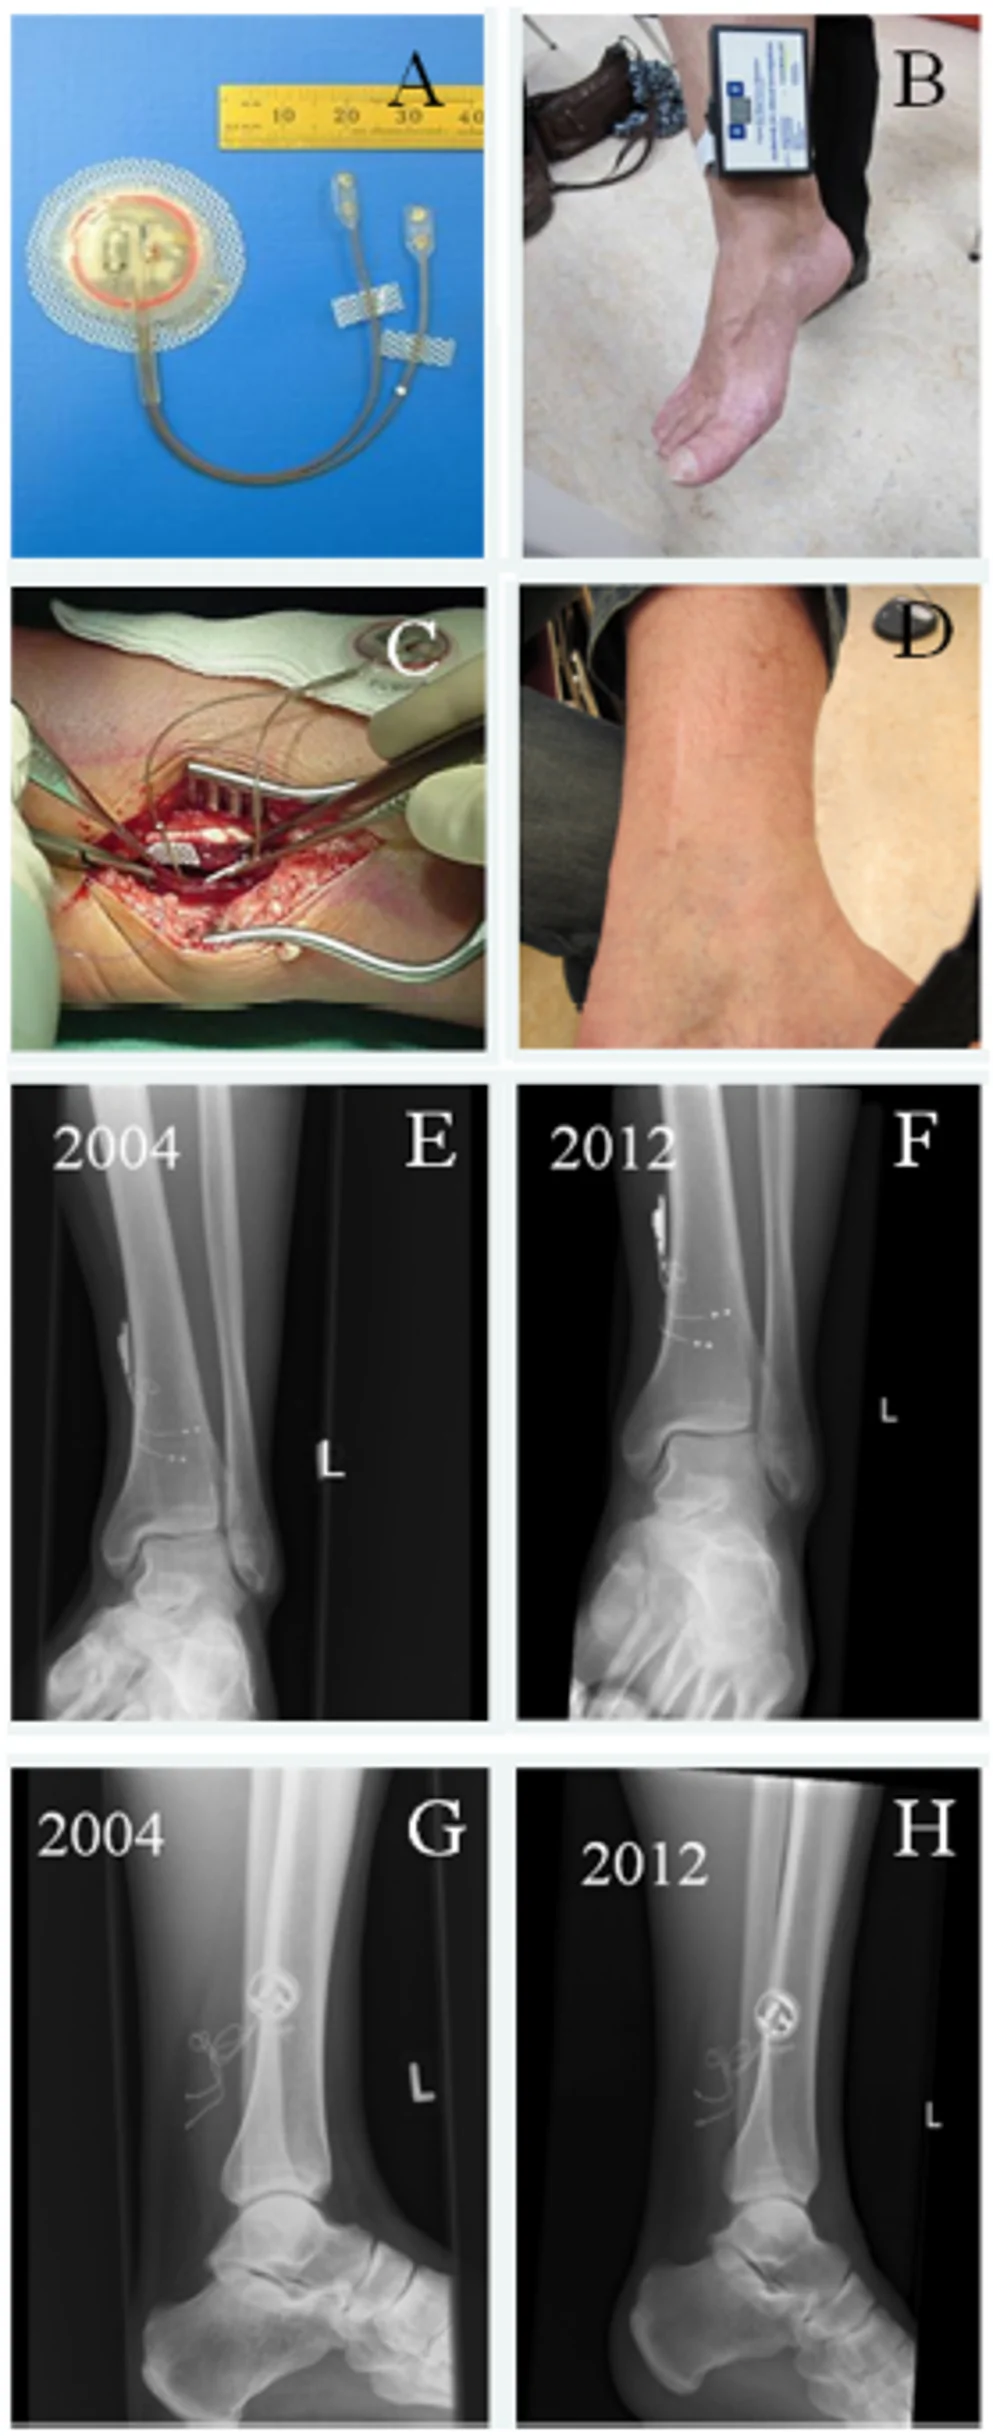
figure reprinted from Janssen et al. [24]. A Urgent-SQ implant consisting of a receiver plate and two electrodes positioned adjacent to the posterior tibial nerve. B External ankle-worn pulse generator used for on-demand stimulation. C Minimally invasive implantation procedure with subcutaneous placement of the receiver plate. D Ankle scar 9 years after implantation. E–H X-ray ankle, anteroposterior and lateral ankle view obtained in 2004 and 2012 demonstrating intact implants without migration, displacement or deformations after 9 year follow-up [24]
